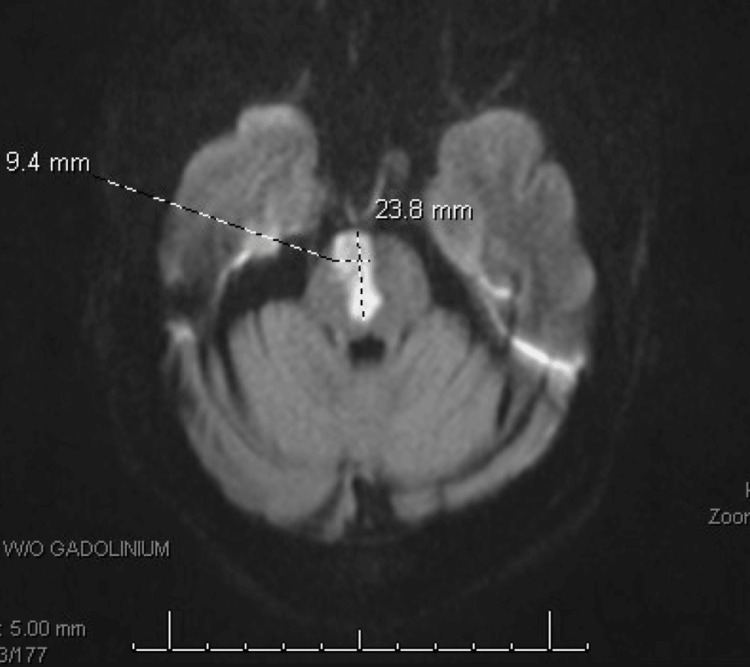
MRI of the brain without contrast demonstrating an acute infarct in the right paramedian pons.
